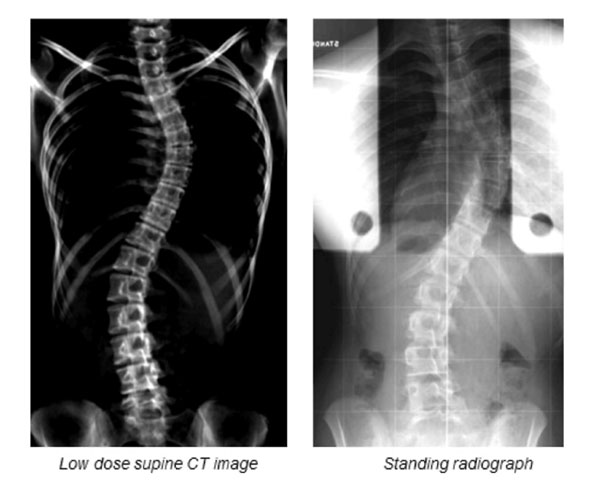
An example of a reformatted supine CT image Cobb 46° (left) and the corresponding standing radiograph Cobb 58° (right)
